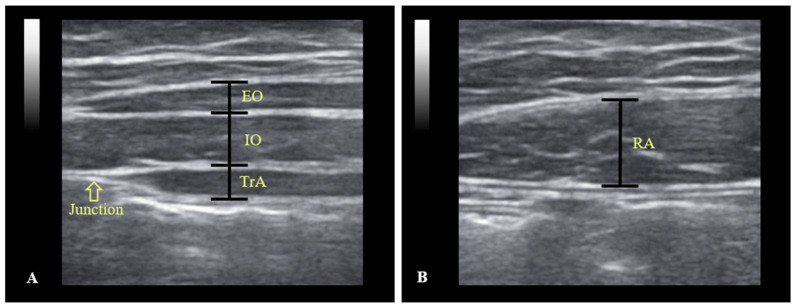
Example ultrasound images of the abdominal muscles. (A) Ultrasound image of external oblique (EO), internal oblique (IO) and transversus abdominis (TrA). (B) Ultrasound image of rectus abdominis (RA).
